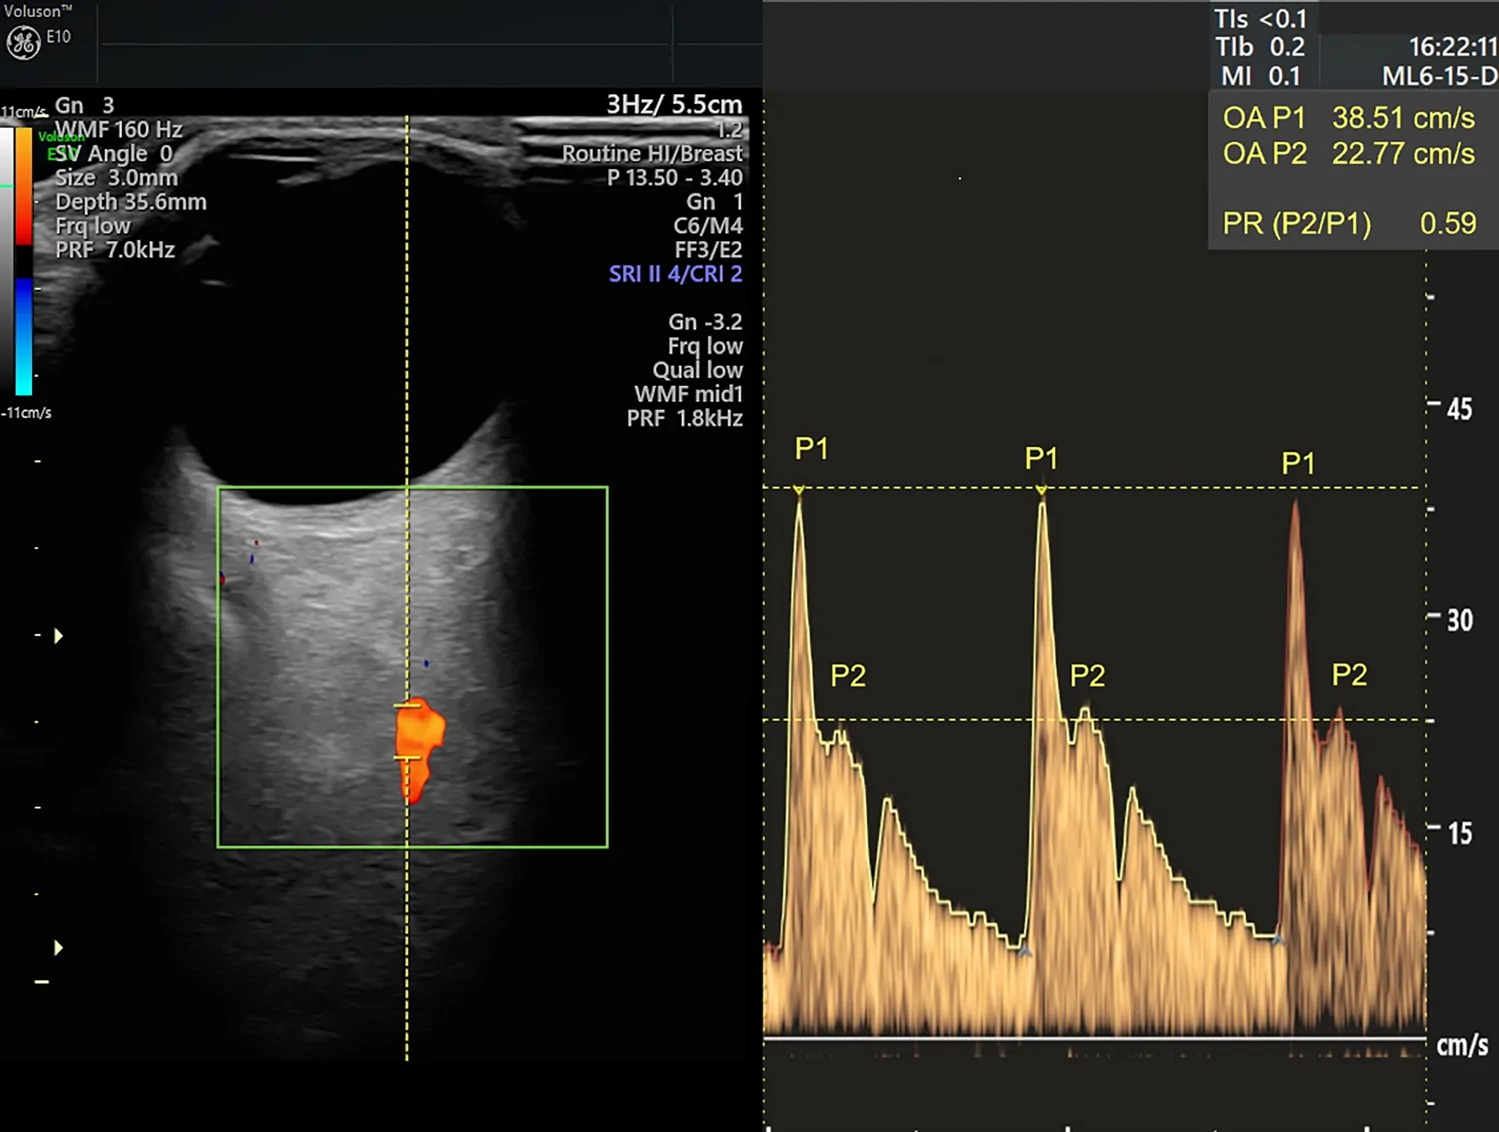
Sonography of the ophthalmic artery with corresponding flow velocity waveform. Left: identification of the OA medial to the optic nerve by color flow mapping (left). Right: typical OA-Doppler sonogram showing a biphasic systole with two systolic peaks, P1 and P2 (prior to the dicrotic notch)
